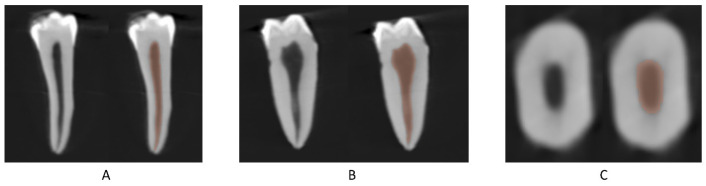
Frontal (A), cross-section (B) and axial (C) CBCT view of an initial premolar with and without the segmentation of the canal.
